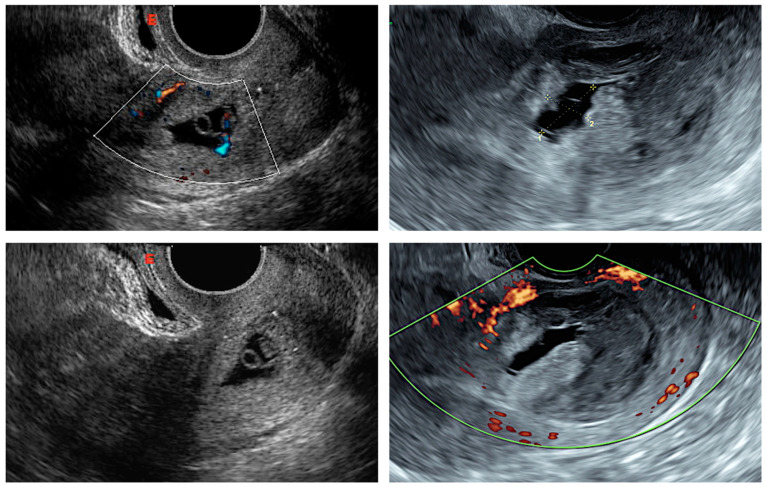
Transvaginal sonographic views of cesarean scar pregnancy.
